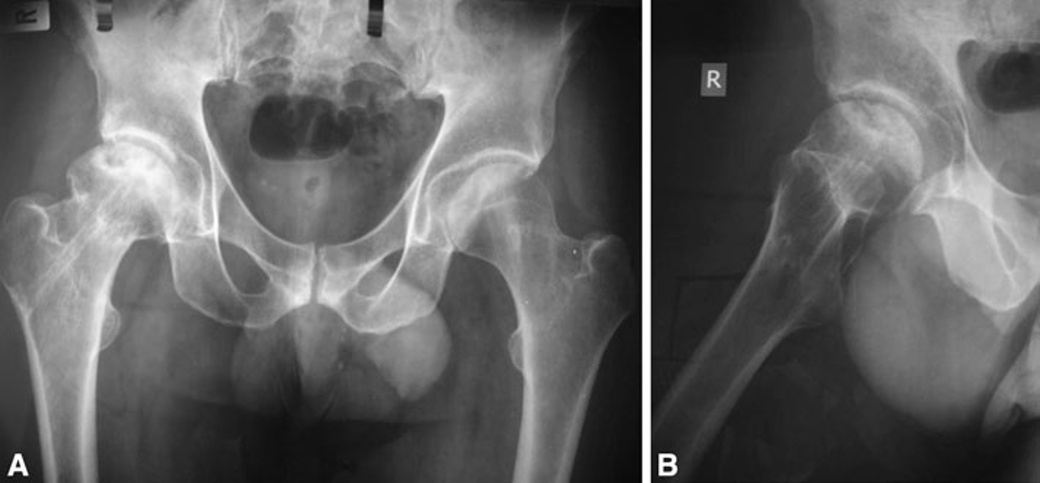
a Anteroposterior and b lateral radiographs of the right hip joint show avascular necrosis of the femoral head in a 70-year-old man 3.5 years after closed reduction and internal fixation for a Garden type III femoral neck fracture
